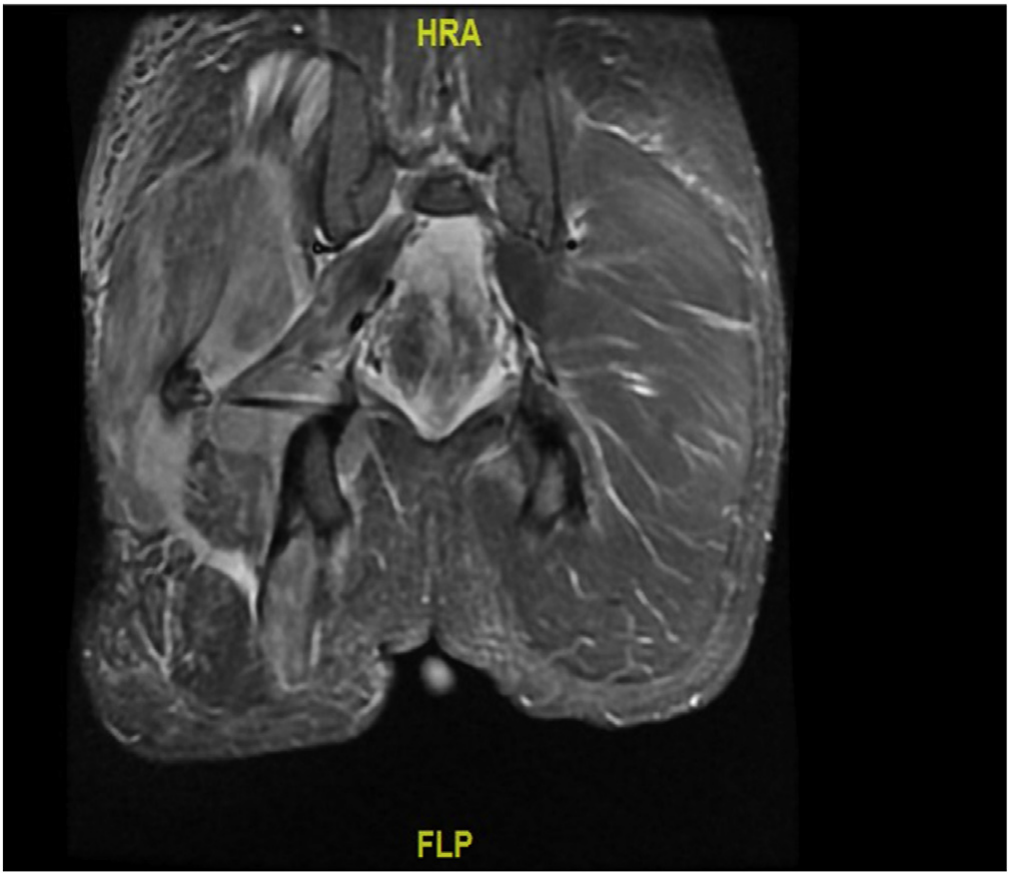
MRI Showing Right hip musculature and intermuscular fascial edema in the right hip: characteristics for post-traumatic changes.
